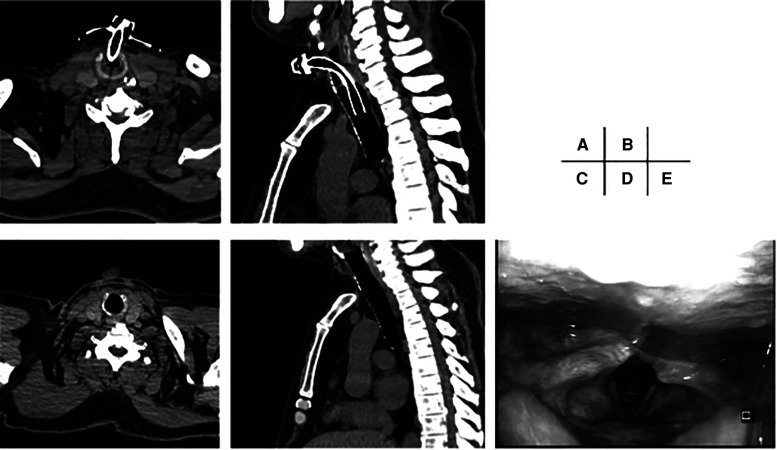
Ct scans and endoscopic image of larynx before and after decannulation. (A,B): CT scan before decannulation. The cannula is inserted at the level of the cricoid cartilage. (C,D): CT scan three months after decannulation. No stenosis is observed. (E): Endoscopic image of larynx three months after decannulation. No laryngeal stenosis is observed.
